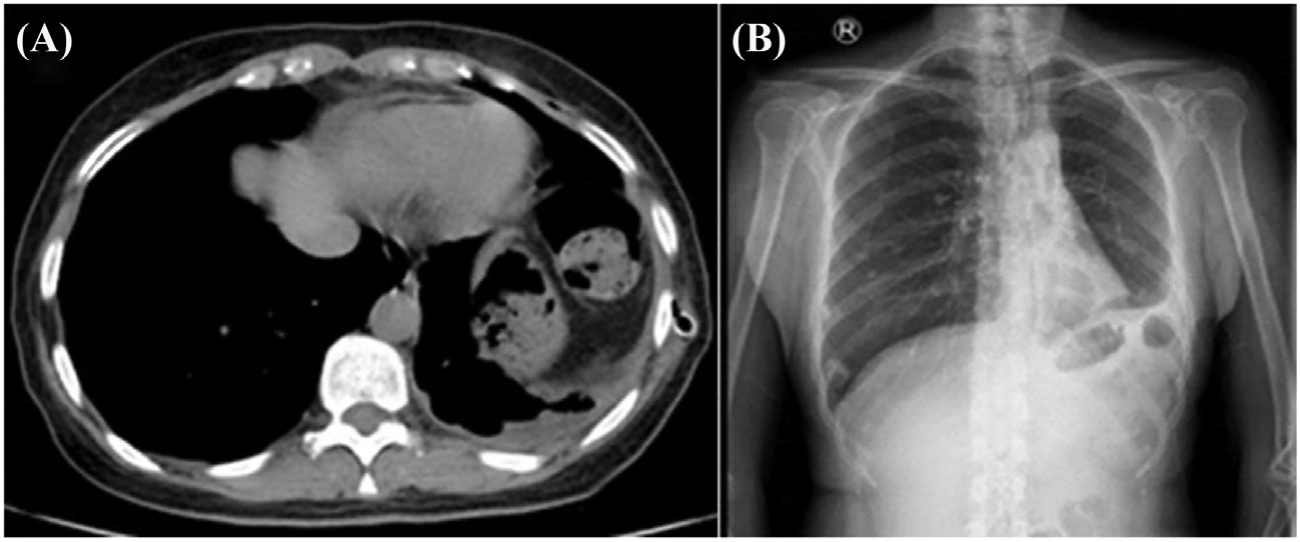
(A) CT and (B) chest X‐ray images of the patient undergoing left lower lobectomy.
